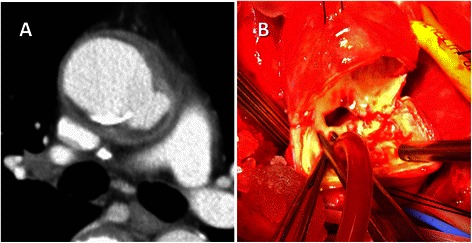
a Computed tomography showed a pseudoaneurysm and abscess in the atherosclerotic lesion of the ascending aorta (Case 1). b There was a large purulent pericardial effusion. The abscess extended to just above the main trunk of the left coronary artery, and the aorta was “punched out”. The cultures of both pericardial effusion and resected aorta were positive for methicillin-sensitive *Staphyrococcus aureus*.”
